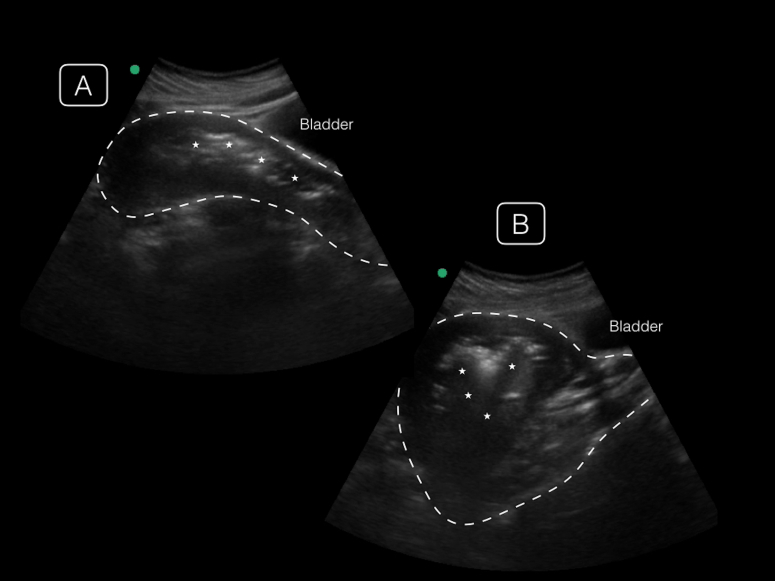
Point-of-care ultrasonographic transabdominal images of the uterus in midline (A) and off-axis sagittal views (B), demonstrating an enlarged uterus (dotted line) with irregular echogenic endometrial debris (asterisk) casting shadows from the endometrium.
